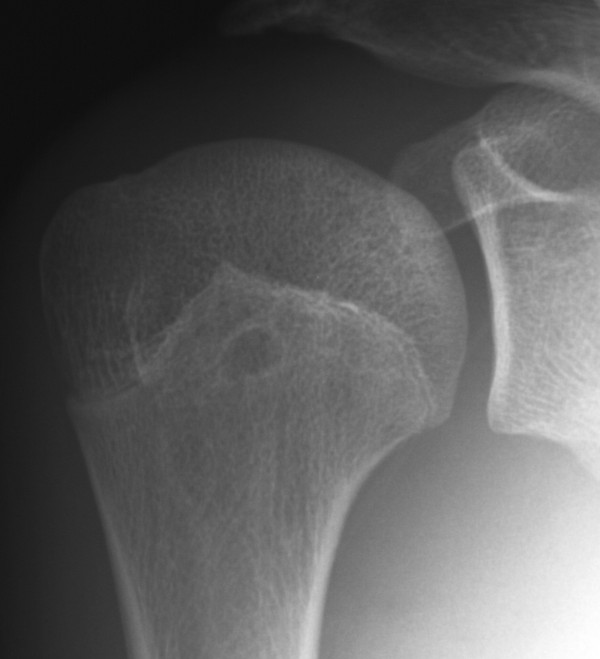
An anteroposterior plain radiograph (2009-3-31) shows the lesion had been totally healed.
